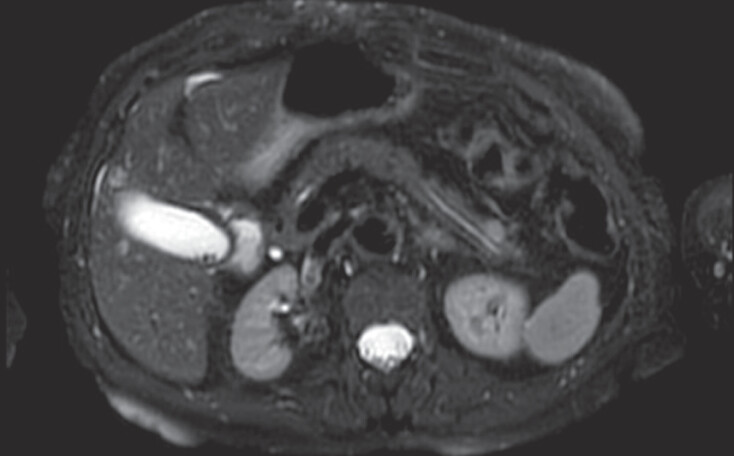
The T2-weighted MRI appearance of the solid pancreatic
